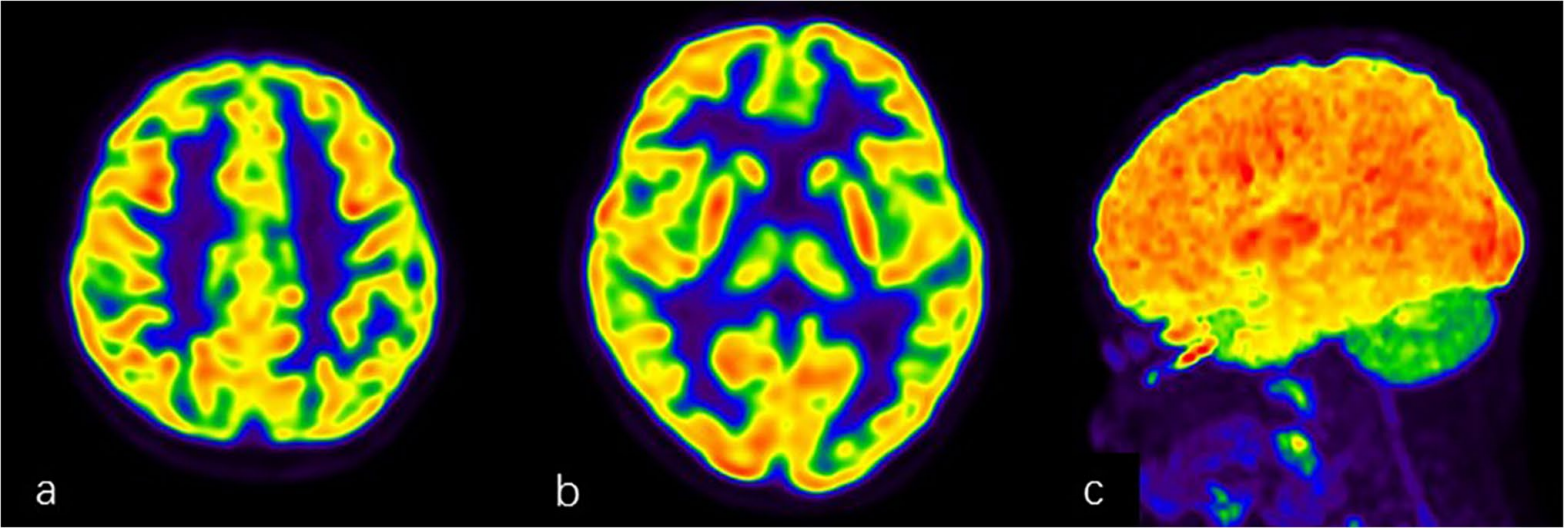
A 7-year-old boy with a 5-year history of seizures presented with normal brain ^18^F-FDG PET/CT imaging. a, b Cross-sectional image of FDG PET. c:MIP map of FDG PET
